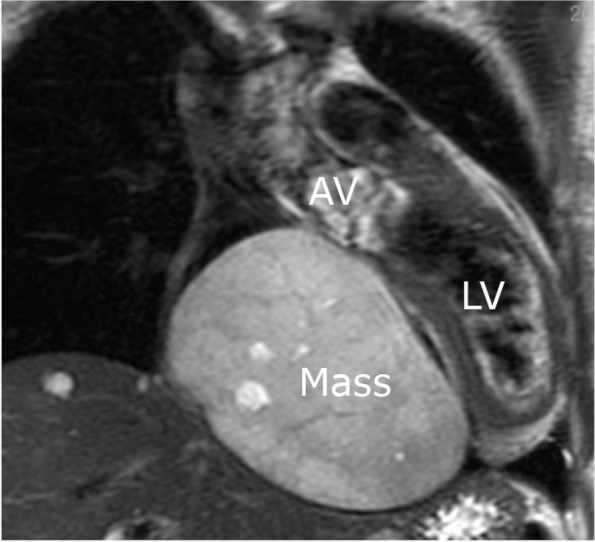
A magnetic resonance image on T2 (coronal plane)-weighted image showing the tumor has a high signal intensity. The mass is compressing LV toward the left side, with no significant evidence of infiltration. AV, aortic valve; LV, left ventricle
